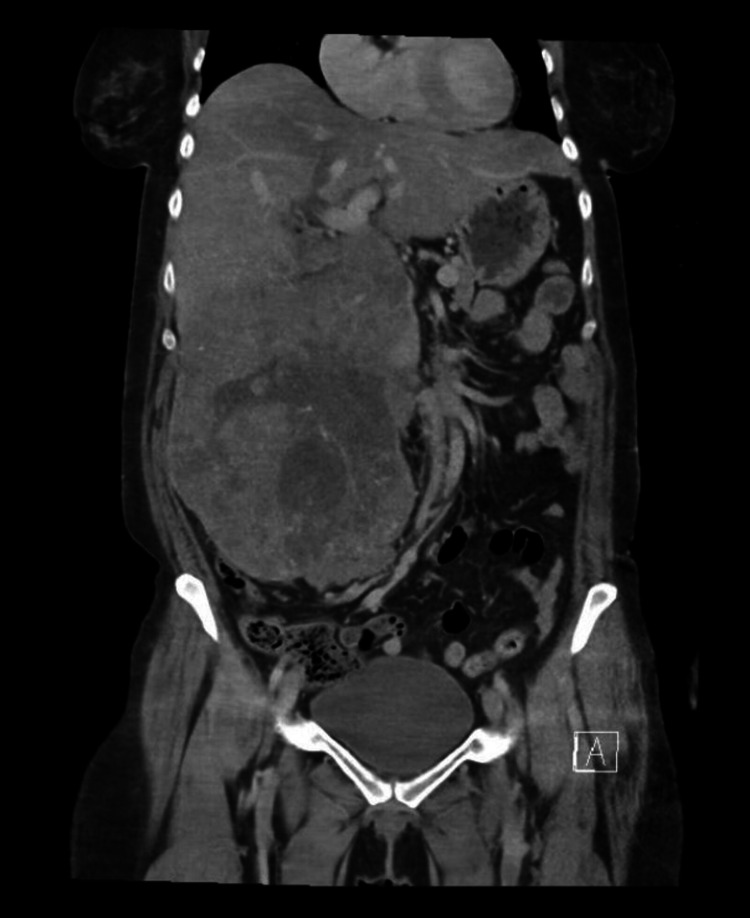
Abdominal CT with contrast in coronal plane showing a very large tumor in right mid-to-lower abdomen arise from the right kidney, which is being totally replaced. The mass is at least 20.1 x 15.6 x 23.7 cm with tumor thrombus extending into the inferior vena cava. Grossly there is no hepatomegaly, ascites, or metastasis in the liver.
